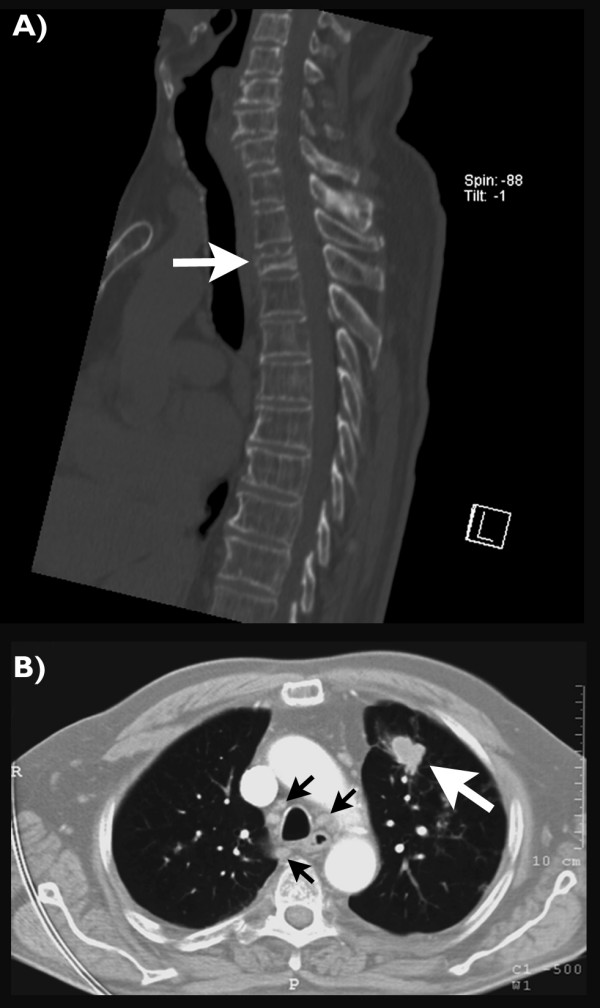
CT scans of the spine and the chest. The sagittal reconstruction of the native CT scan of the spine (A) confirms a compression fracture of the third thoracic vertebra with lytic destructions of posterior margins of both adjacent vertebral bodies (arrow). The axial reconstruction of the contrast enhanced CT scan of the chest (B) suggests a tumorous lesion of the ventral left upper lobe (large arrow) with accompanying mediastinal lymphadenopathy (small arrows).
